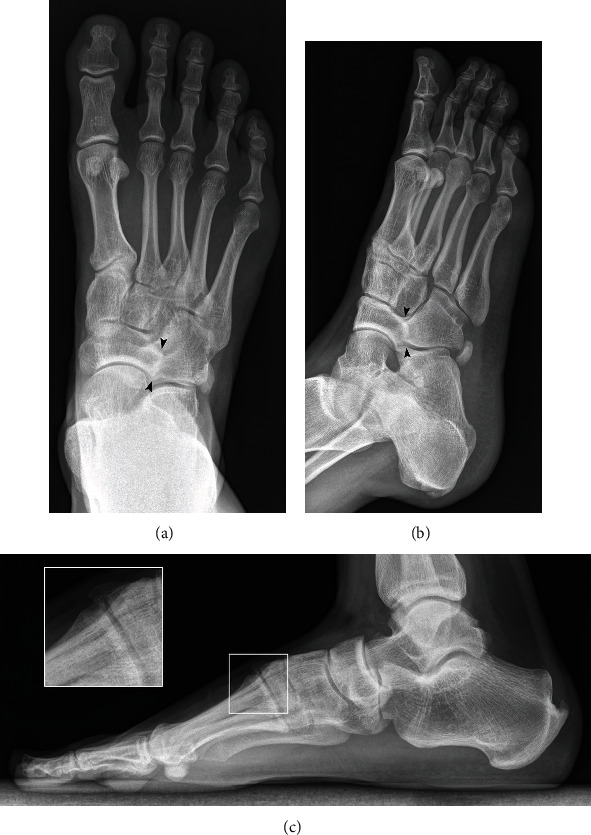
Frontal (a) and oblique (b) x-ray image of the right foot show an osseous cubonavicular coalition (arrowheads) with an absence of joint space. Lateral (c) x-ray image of the right foot shows degenerative changes with a spiky osteophytic deformation on the dorsal aspect of the first tarsometatarsal joint and a joint line irregularity (side shot).
